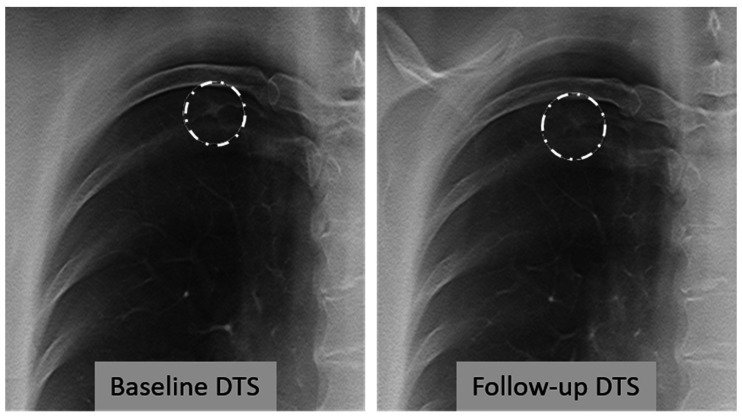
Example of a nodule located outside the focal plane on follow-up. A 59-year-old woman, previous smoker (11.5 pack-years), exposed to cigarette smoke at work, no history of cancer. Incidental, solid nodule in the right upper lobe. Automated measurements on CT provided volume and average diameter of 110 mm^3^ and 7 mm on baseline and 141 mm^3^ and 8 mm on follow-up, and a volumetric change between the examinations of +28% and a VDT of 1632 days. Average diameter base on the two diameters on DTS were 6 mm on baseline for both observers, and 3 and 4 mm on follow-up, and a calculated volumetric change of –76% and –67% for the two observers. The nodule appears to be in the focus plane on baseline DTS, but outside the plane on follow-up. CT, computed tomography; DTS, digital tomosynthesis; VDT, volume doubling time.
